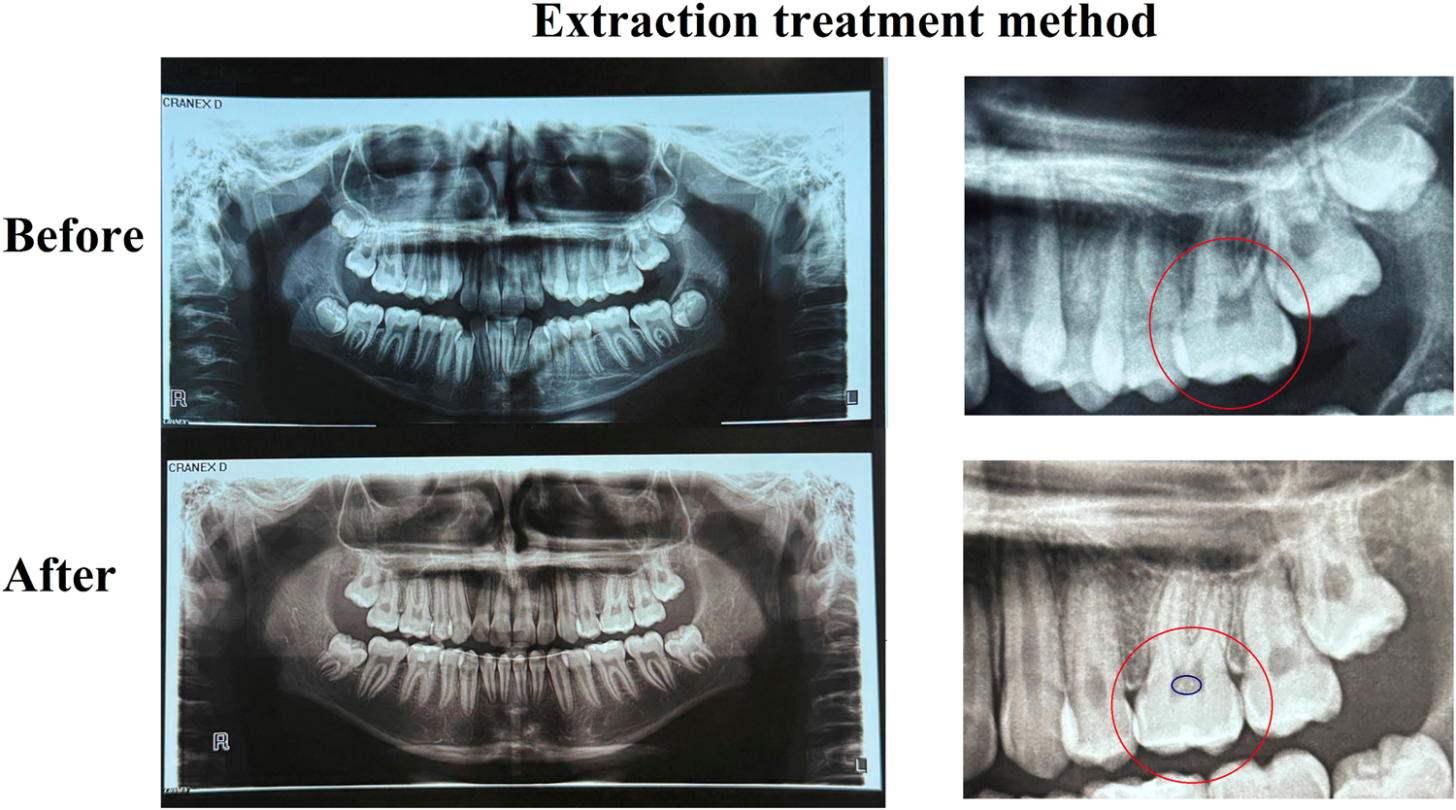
Pre‐ and post‐treatment radiographs of a patient who underwent fixed orthodontic treatment with the extraction method. The red circle highlights the tooth that developed a pulp stone, while the blue circle indicates the location of the pulp stone(s).
